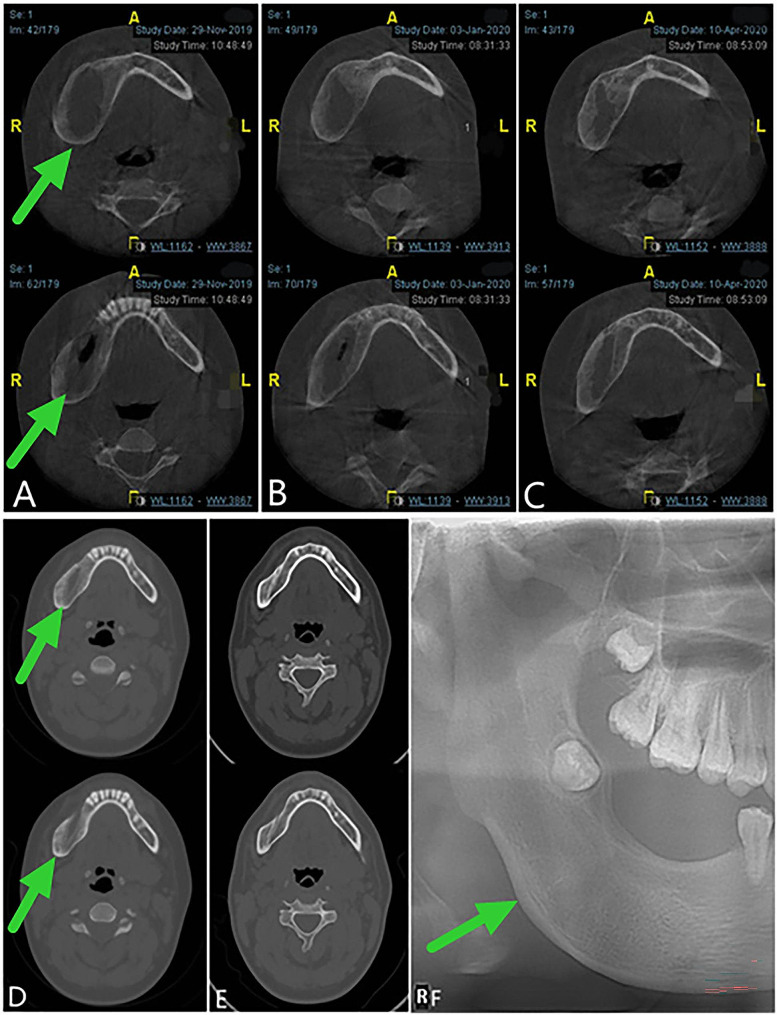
Follow-up images. A, B and C, Cone beam computed tomography showing 5, 7,10 months respectively after the second operation. D and E, Computed tomography view displaying the continuous osteogenic remodeling 4 and 15 months after the window is closed. F, Panoramic oral radiogram demonstrating 15 months after the surgery to close the window
